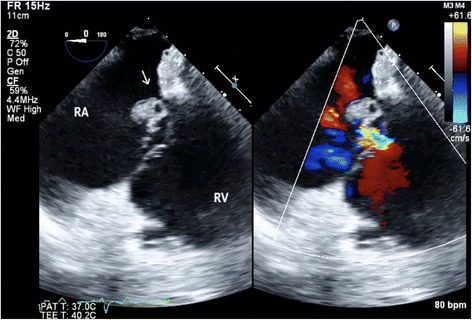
Transesophageal echocardiography (TEE) in the mid-esophageal modified four-chamber view. *Left* two-dimensional TEE, demonstrating the vegetation attached to the annulus of the aortic valve (*arrow*). *Right* color Doppler TEE, demonstrating the abnormal flow into the right atrium
